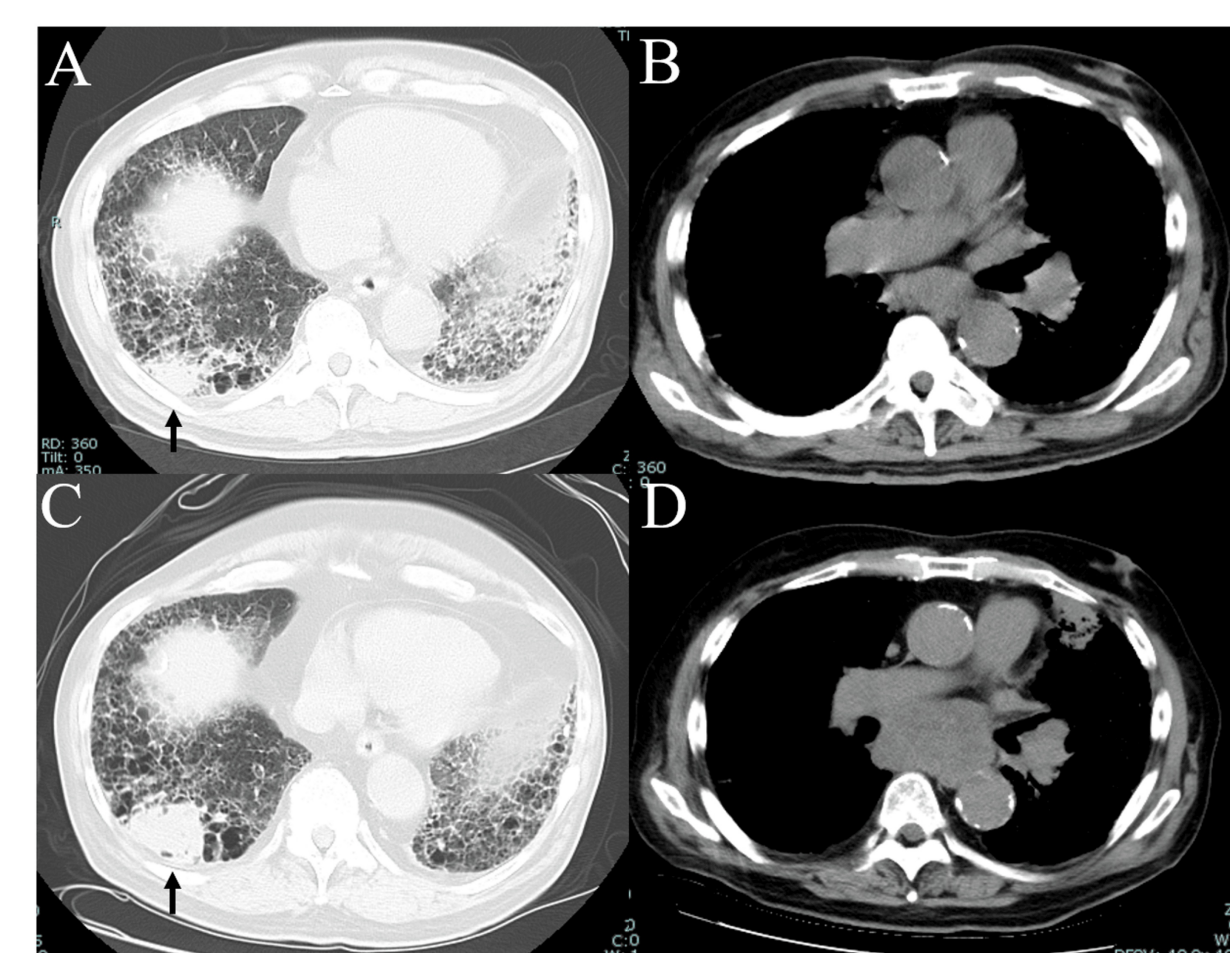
Computed tomography showing a lung mass and enlarged mediastinal lymph nodes at different time points. (A) and (B) were taken 12 months after the diagnosis of the second cancer, with a newly developed lung mass in the right lower lobe, while (C) and (D) were captured 24 months post-diagnosis. Images A and C depict the lung mass (arrows), and B and D show the subcarinal region.
